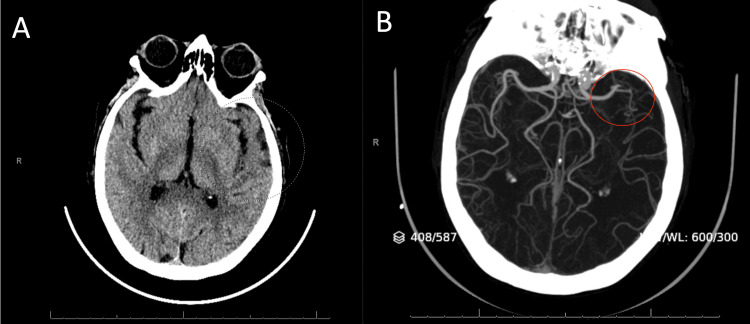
CT scans of the head following stroke in our patient A) Non-contrast CT scan and B) CT angiogram
